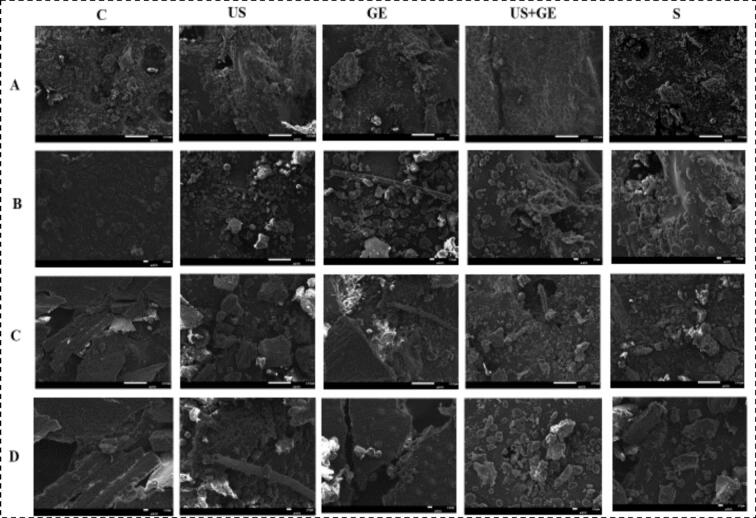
Morphological characterization of highland’s barley fractions subjected to ultrasonication, germination, ultrasound assisted germination and soaking. A. whole barley flour 200X. B. Whole barley flour 400X. C. Whole barley bran 200X D. Whole barley bran 400X. C: untreated barley, US: multifrequency ultrasonication, GE: germination, S: soaking.
